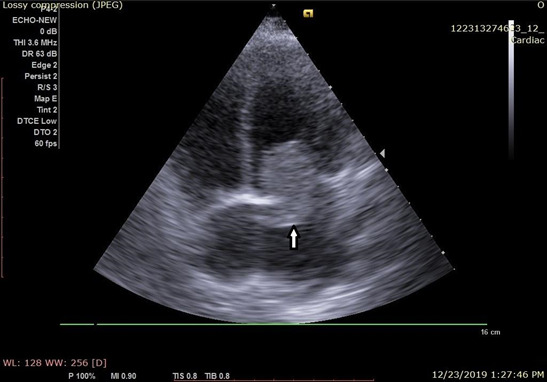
Transthoracic echocardiography revealed a mass arising from inter-atrial septum in the left atrium, and prolapsing towards left ventricle, suggestive of left atrial myxoma (indicated by arrow).
